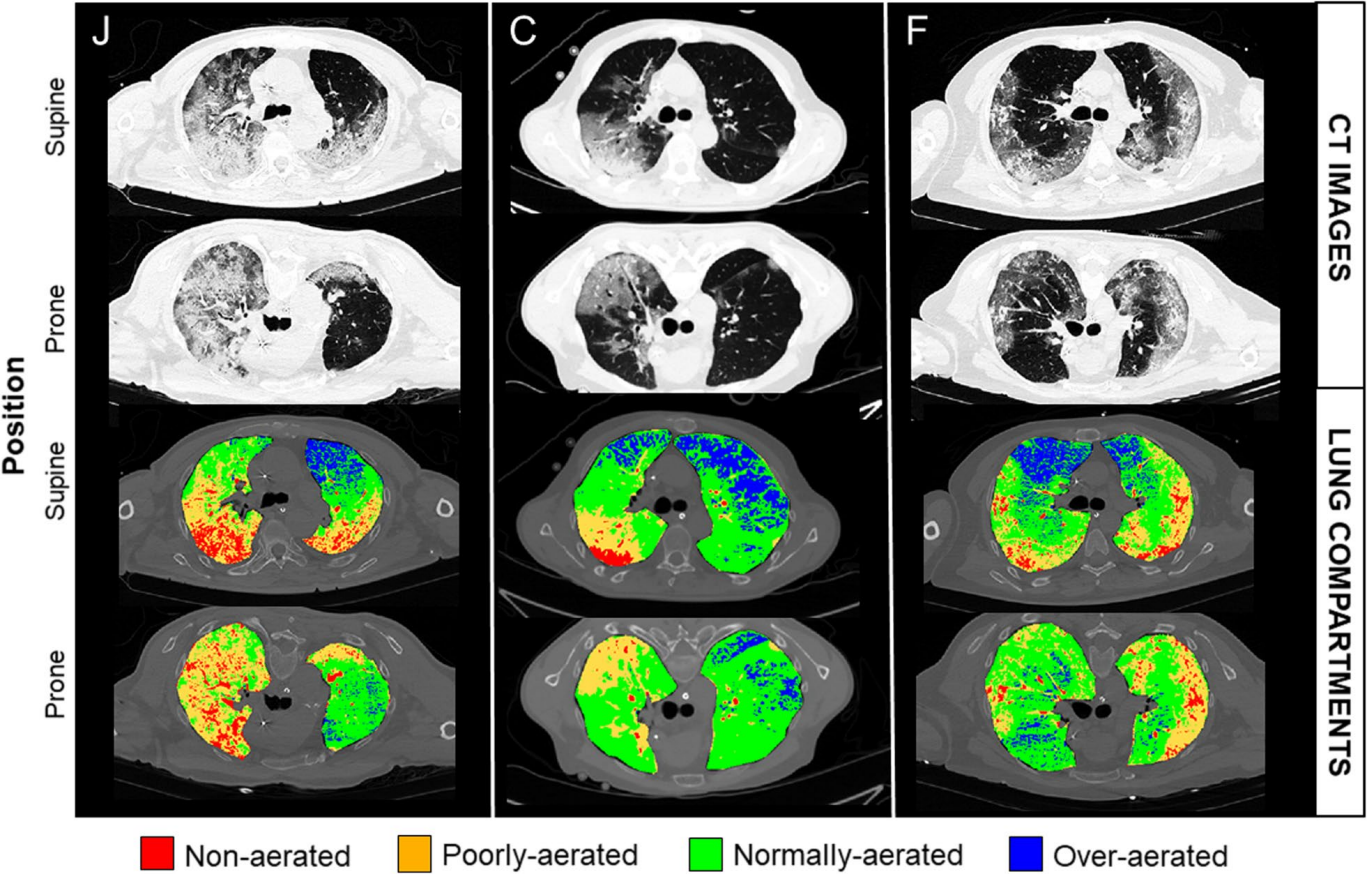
Colour-coded analysis of lung computed tomography (CT) data. Representative CT images taken at the level of carina from three patients with COVID-19 in the supine and prone position, with a very large decrease in the volume of the over-aerated compartment in response to prone positioning. Upper panels: original lung CT images, with aeration shown on a continuous grayscale. Lower panels: using an automated encoding system, we attributed a specific colour to the non-aerated, poorly-aerated, normally-aerated, and over-aerated compartments. The three patients are identified with the same letters as in other figures. With prone positioning, the volume of the over-aerated lung decreased from 318 to 121 ml in patient J; from 738 to 148 ml in patient C; from 503 to 230 ml in patient F
